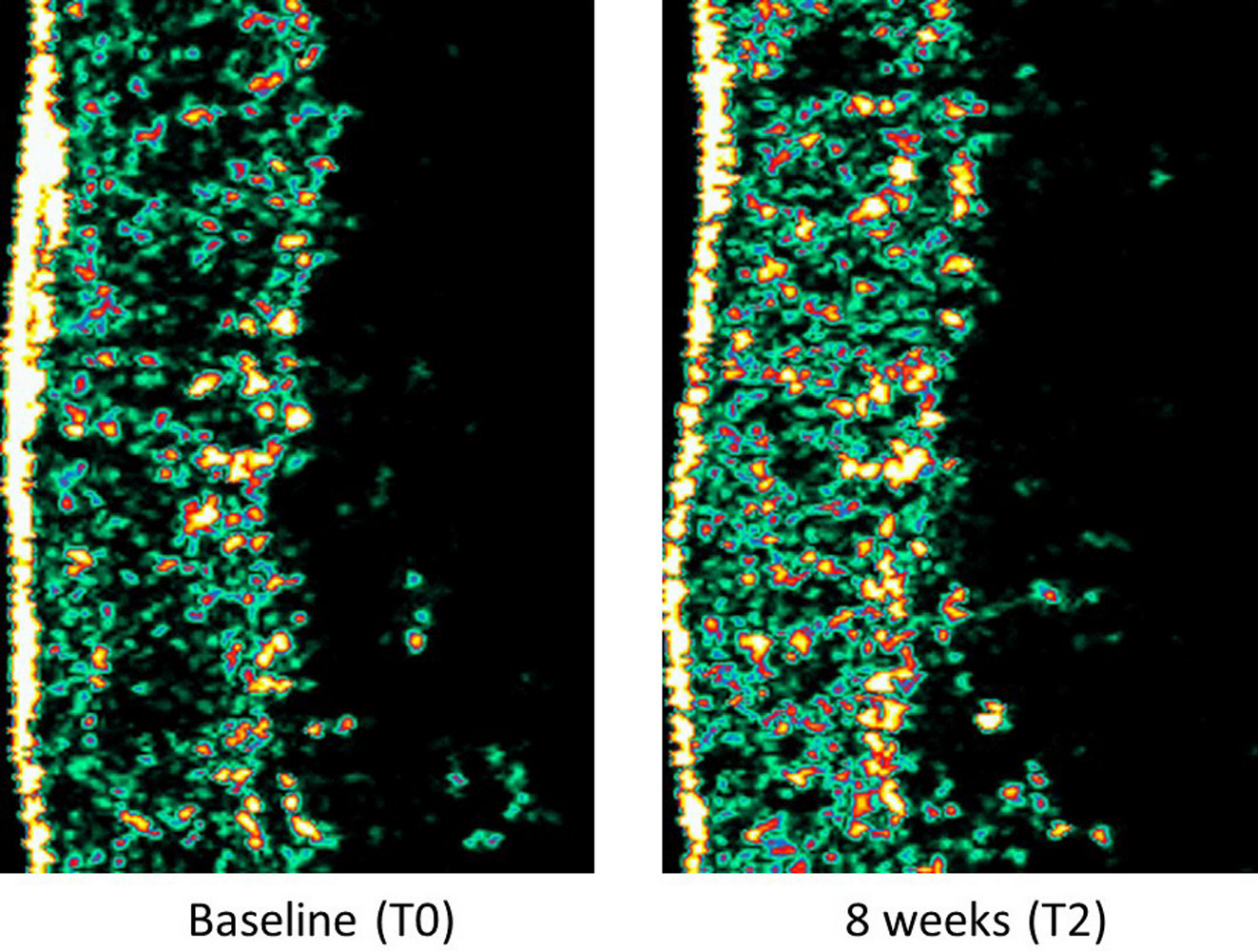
The high‐frequency ultrasonography images of the cross‐sectional view of the skin at baseline (T0) and after ingestion of TCP for 8 weeks (T2). Images were obtained using Dermalab^®^ Combo, 20 MHz ultrasound probe (Cortex Technology ApS, Denmark). Colors represent the intensity of the reflected signal from the skin. Different colors in the image correspond to the varying strengths of the ultrasound signal reflection. Dark colors (e.g., fat layer) indicate low levels of reflection, while light colors (i.e., yellow and green) represent stronger reflections. The dermis layer, visualized with a mixture of colors, reflects the diverse tissue compositions within this layer.
